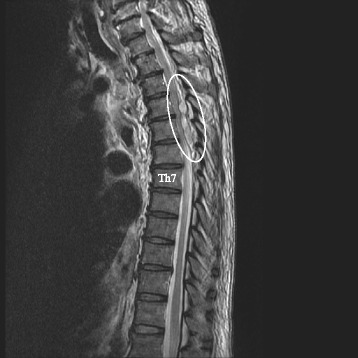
T2-weighted sagittal magnetic resonance image. The spinal epidural hematoma (*circle*) is indicated by the hyperintense region in the epidural space from Th3 to Th6
